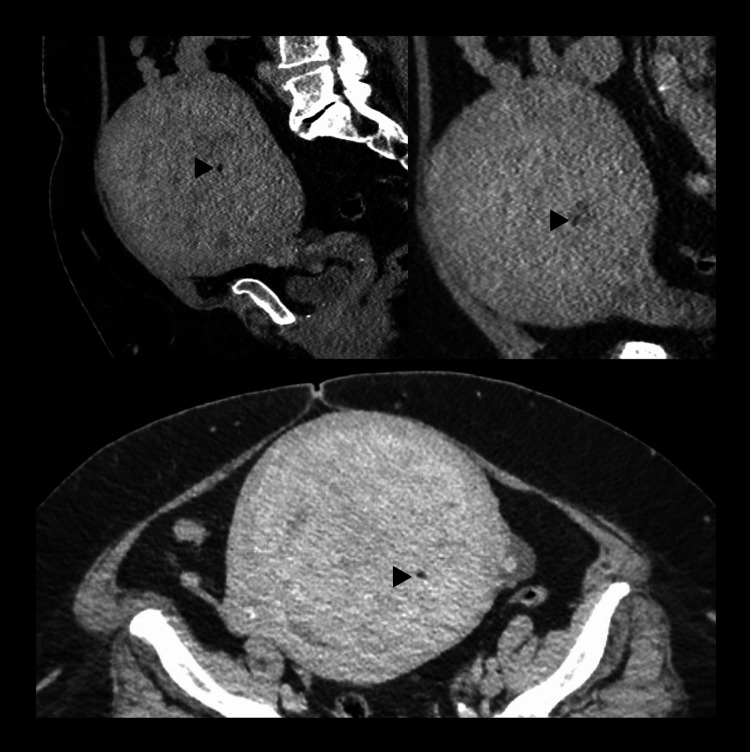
CT scan showing large uterine mass containing small hypodense areas suggestive of lipoleiomyoma (black arrowhead).
